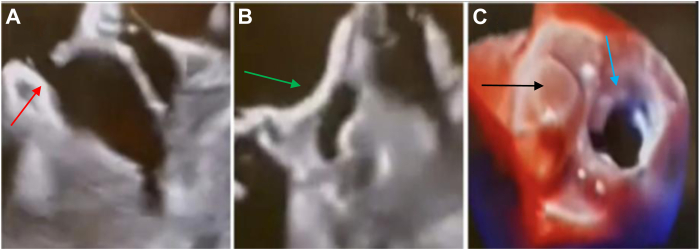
Incomplete surgical LAA closure successfully occluded with Amplatzer Amulet (25 mm). A: Preprocedural TEE demonstrates residual communication through a narrow surgical fenestration (*red arrow*) after previous surgical LAA closure. B: After deployment of a 25 mm Amplatzer Amulet (*green arrow*), TEE shows complete occlusion of the LAA without residual color Doppler flow. C: 3-dimensional TEE at follow-up demonstrates a well-seated device with full coverage of the ostium (*black arrow*), consistent with durable occlusion. The mitral valve can be seen (*blue arrow*). LAA = left atrial appendage; TEE = transesophageal echocardiogram.
